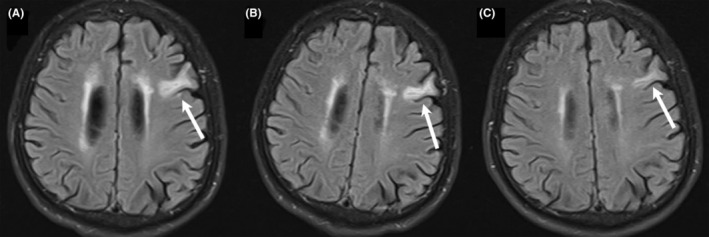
T2 FLAIR images from the patient's cranial MRI evaluation. (A–C) show the images at the time of first diagnosis, the time of recurrence, and the time after the use of OFA for 1 month, respectively.
